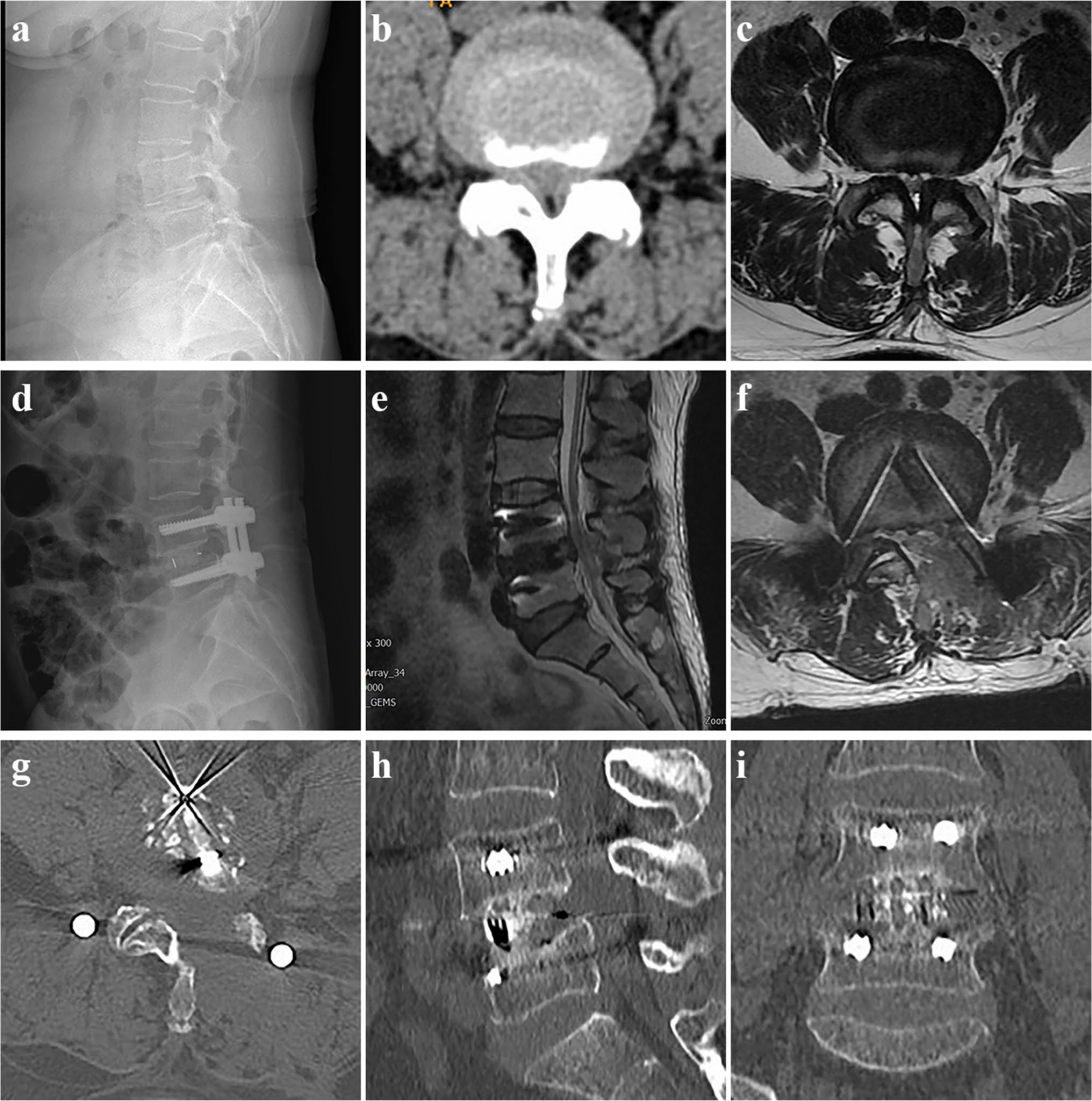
A 58-year-old female with grade 1 spondylolisthesis at L4. a The X-ray indicates grade 1 spondylolisthesis at L4. b–c There is spinal stenosis at the L4–5 level and narrowing of the right neural foramen. d Postoperative X-ray shows a complete reduction of the spondylolisthesis. e–f The postoperative follow-up MRI suggests sufficient decompression of the spinal canal and left lateral recess. g–i Postoperative CT scan showed complete interbody fusion of L4–5 at 6 months after surgery
